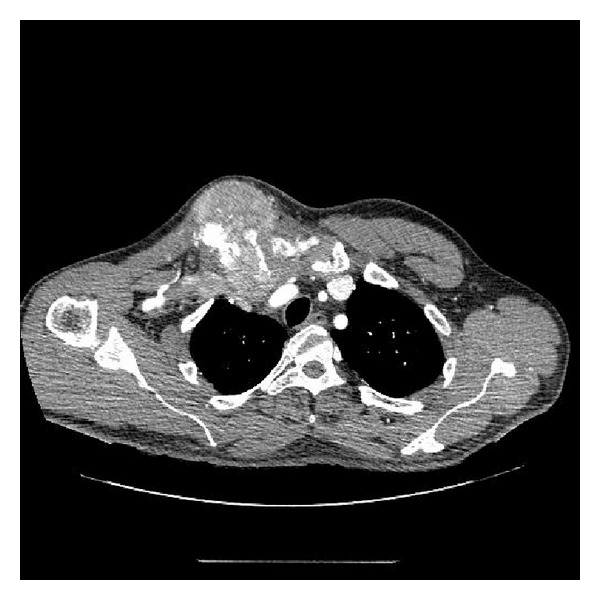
Axial CT section with mass on the medial side of the clavicle.
